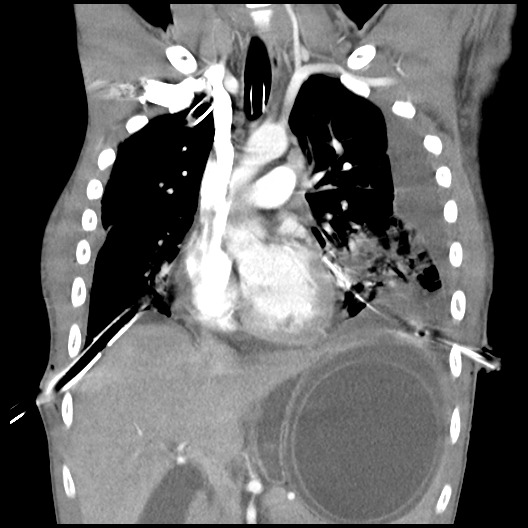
Repeat computed tomography chest scan showing intact splenic cyst and left effusion.
